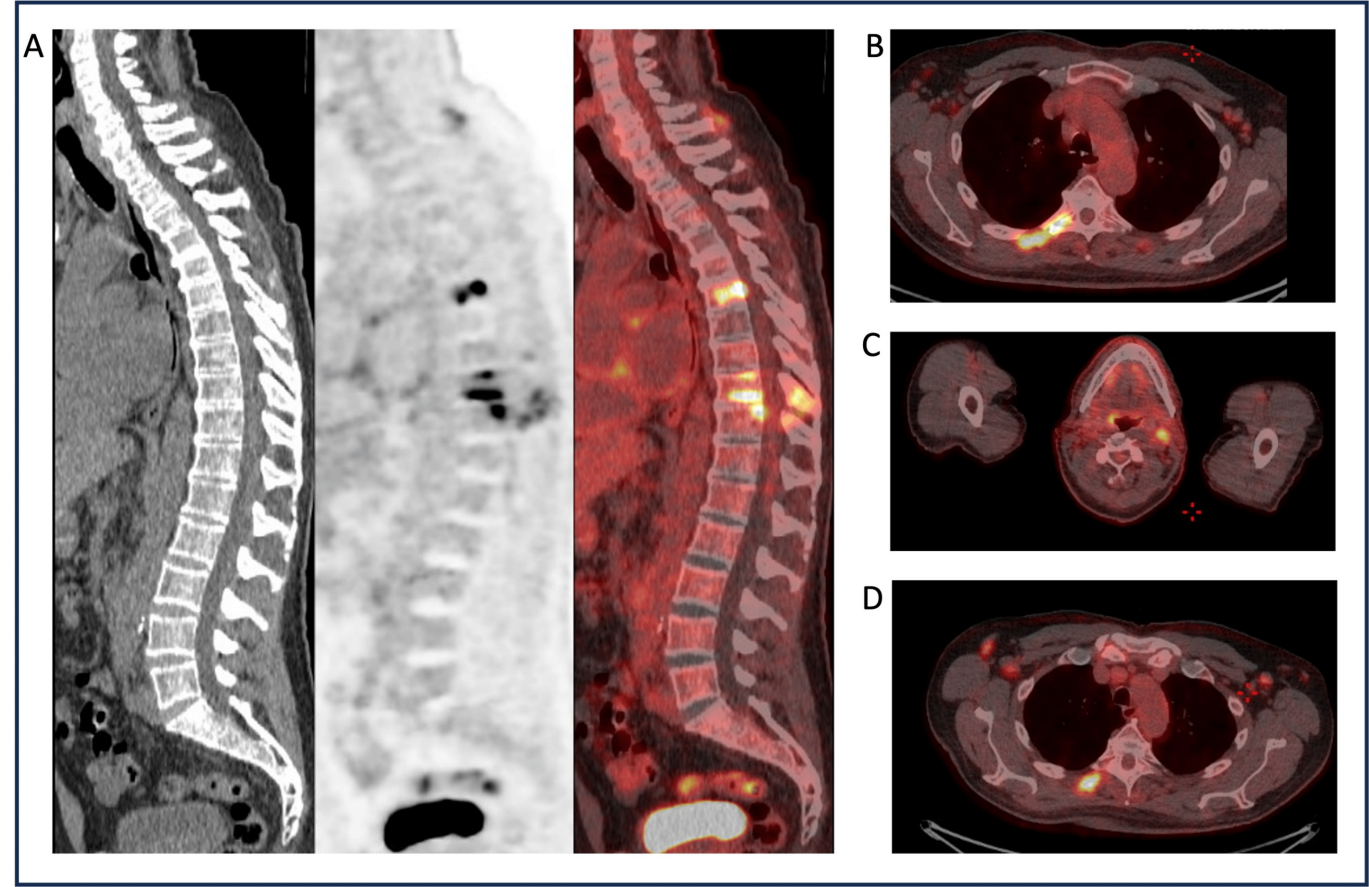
The FDG PET-CT images demonstrating widespread increased uptake in bone and lymph nodes A: Spinal series consisting of (from left to right) CT image, PET image, and fused PET-CT image in sagittal view, demonstrating increased uptake predominantly in thoracic vertebrae; B: Axial view of fused PET-CT image demonstrating increased uptake in ribs; C: Axial view of fused PET-CT image demonstrating increased uptake in cervical lymph nodes; D: Axial view of fused PET-CT image demonstrating increased uptake in axillary lymph nodes anteriorly and ribs posteriorly
